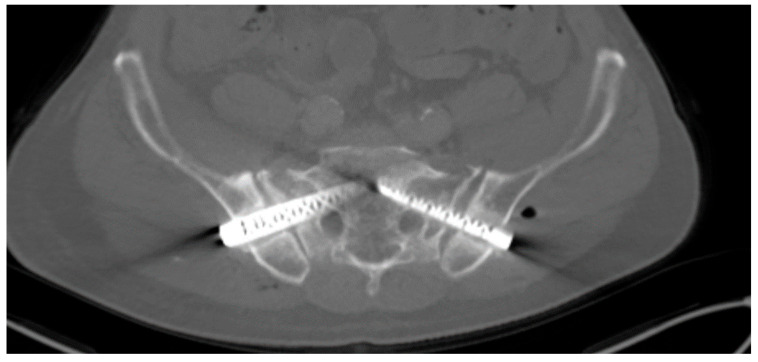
iFuse^®^ implants showing signs of loosening in an axial CT scan prior to removal in a patient who had received delayed SI-joint fusion when insufficiency fractures caused by osteonecrosis had already formed.
